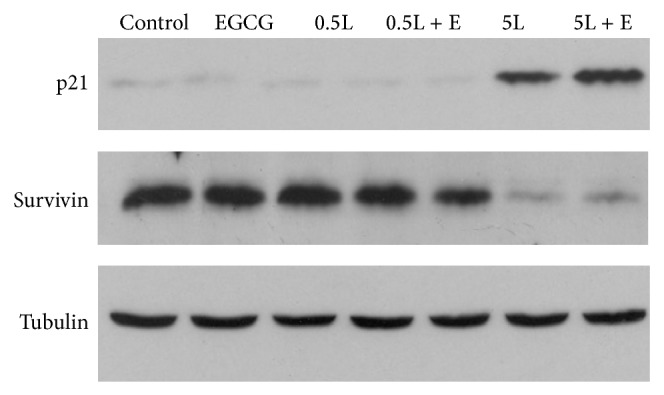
Western blots of p21 and survivin in A549 cells treated for 48 h. Protein was extracted from cells using RIPA lysis buffer. Protein concentration was determined using a Bio-Rad Bradford protein assay. Protein lysate (30 *μ*g) was separated using 12% SDS-PAGE and transferred to a PVDF membrane. After immunoblotting with primary and secondary antibodies, membranes were visualized by chemiluminescence and exposed to X-ray film. E: 20 *μ*M EGCG; 0.5L: 0.5 nM LMB; 5L: 5 nM LMB.
